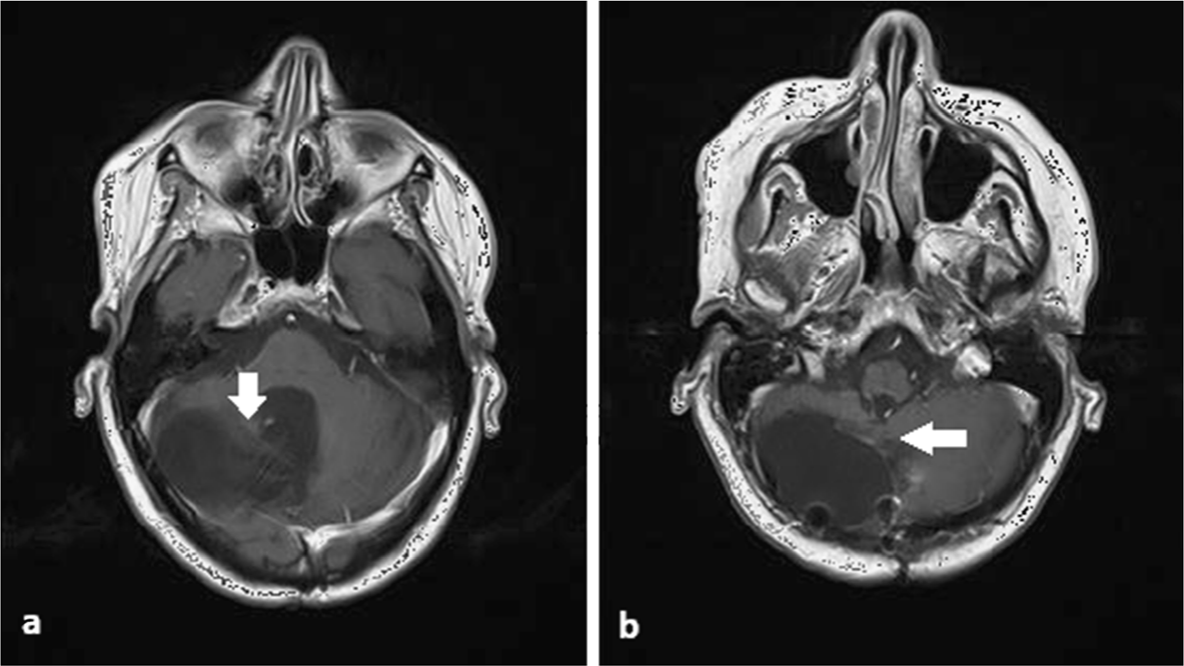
a and b: The magnetic resonance imaging performed at three months after re-irradiation showed complete remission (*white arrows*).
